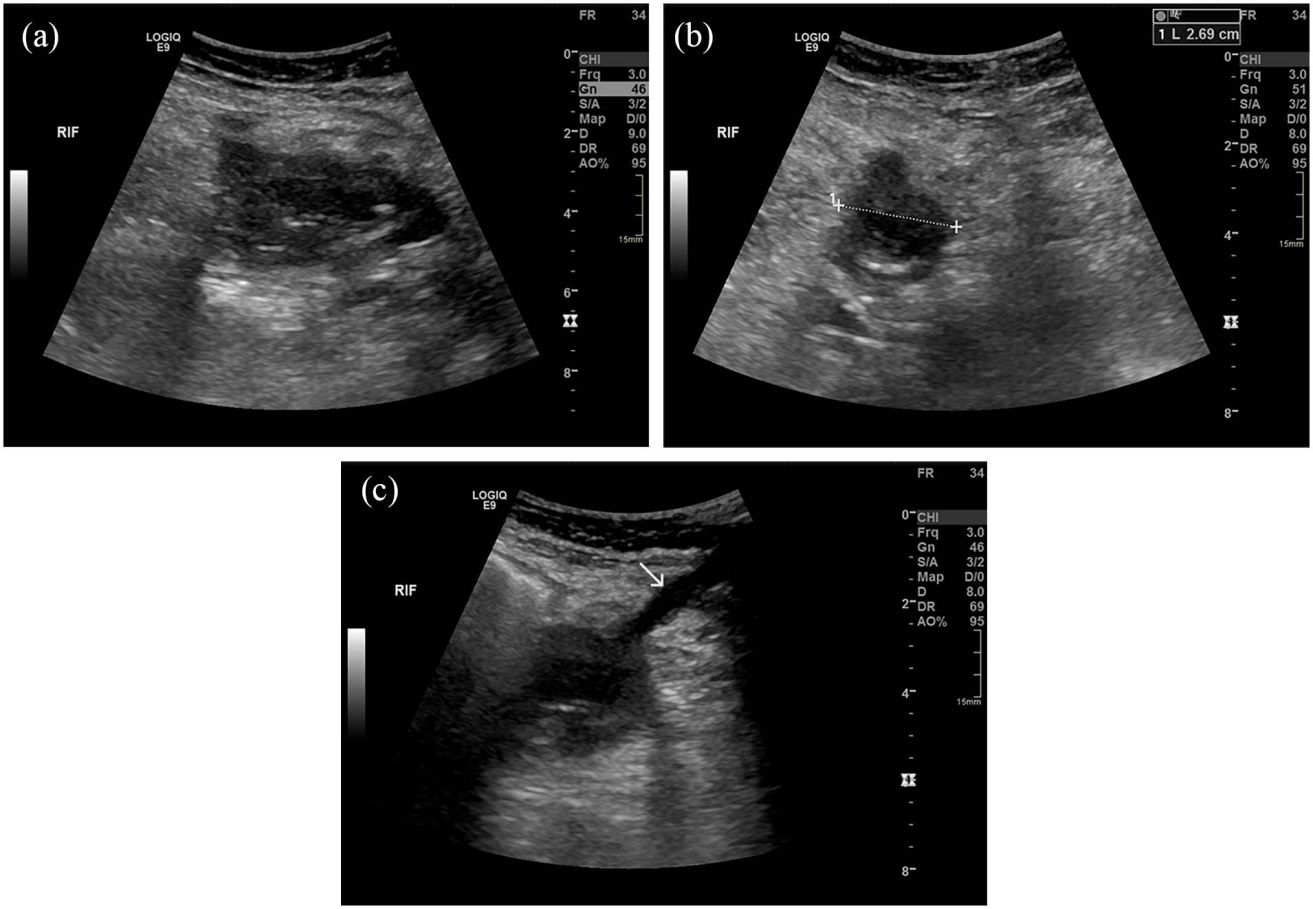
Post-drainage ultrasound: (a) long-section of a remnant complex fluid collection, (b) transverse view, and (c) drain track (arrow).
